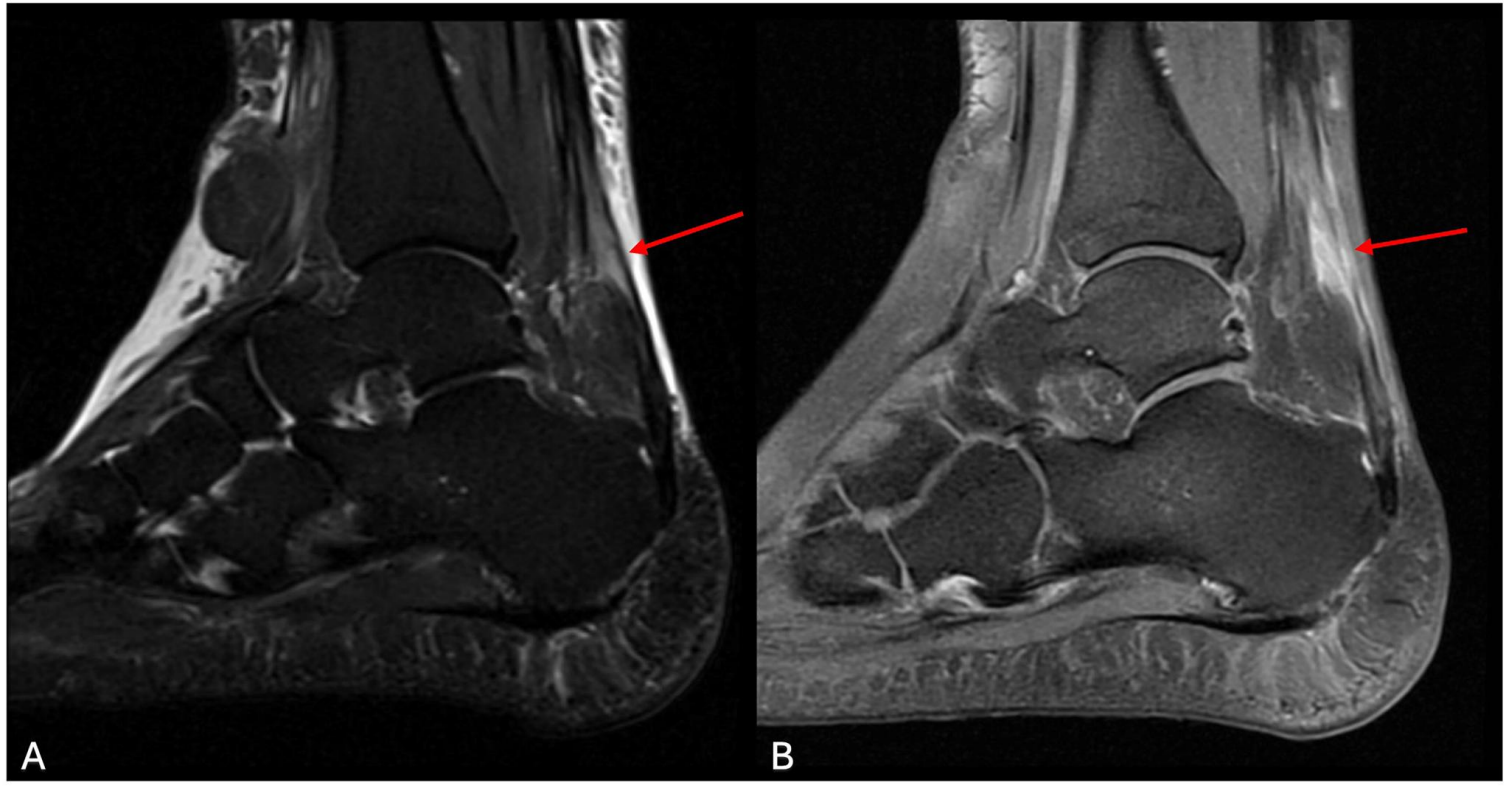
(A) T2 sagittal and (B) T1 post-contrast MRI show chronic disruption of the left Achilles tendon (red arrows).
